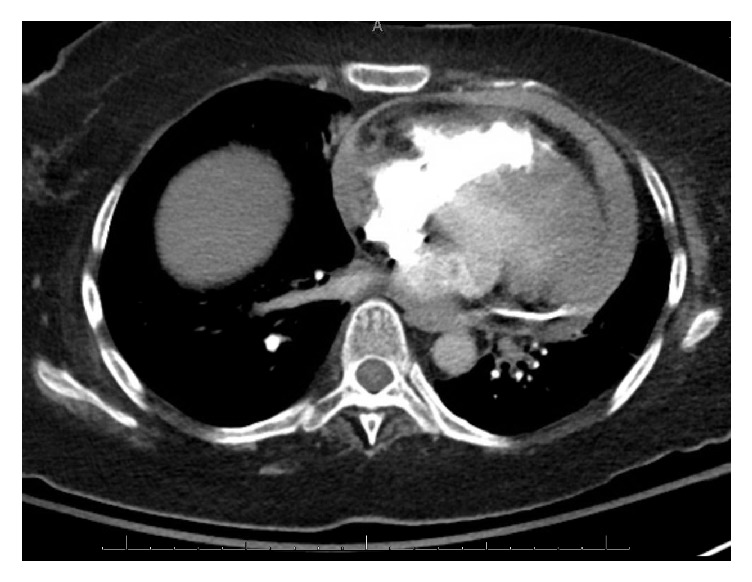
CT scan transverse view with hyperdense pericardial effusion.
